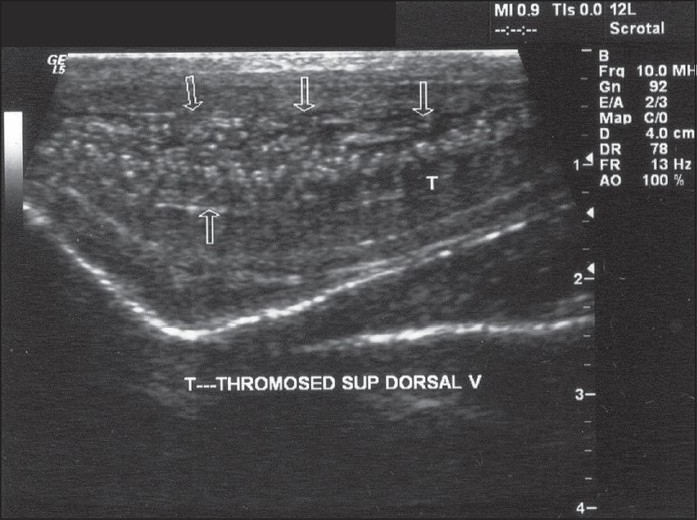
Ultrasound showing thrombosis of the superfi cial dorsal vein of the penis
